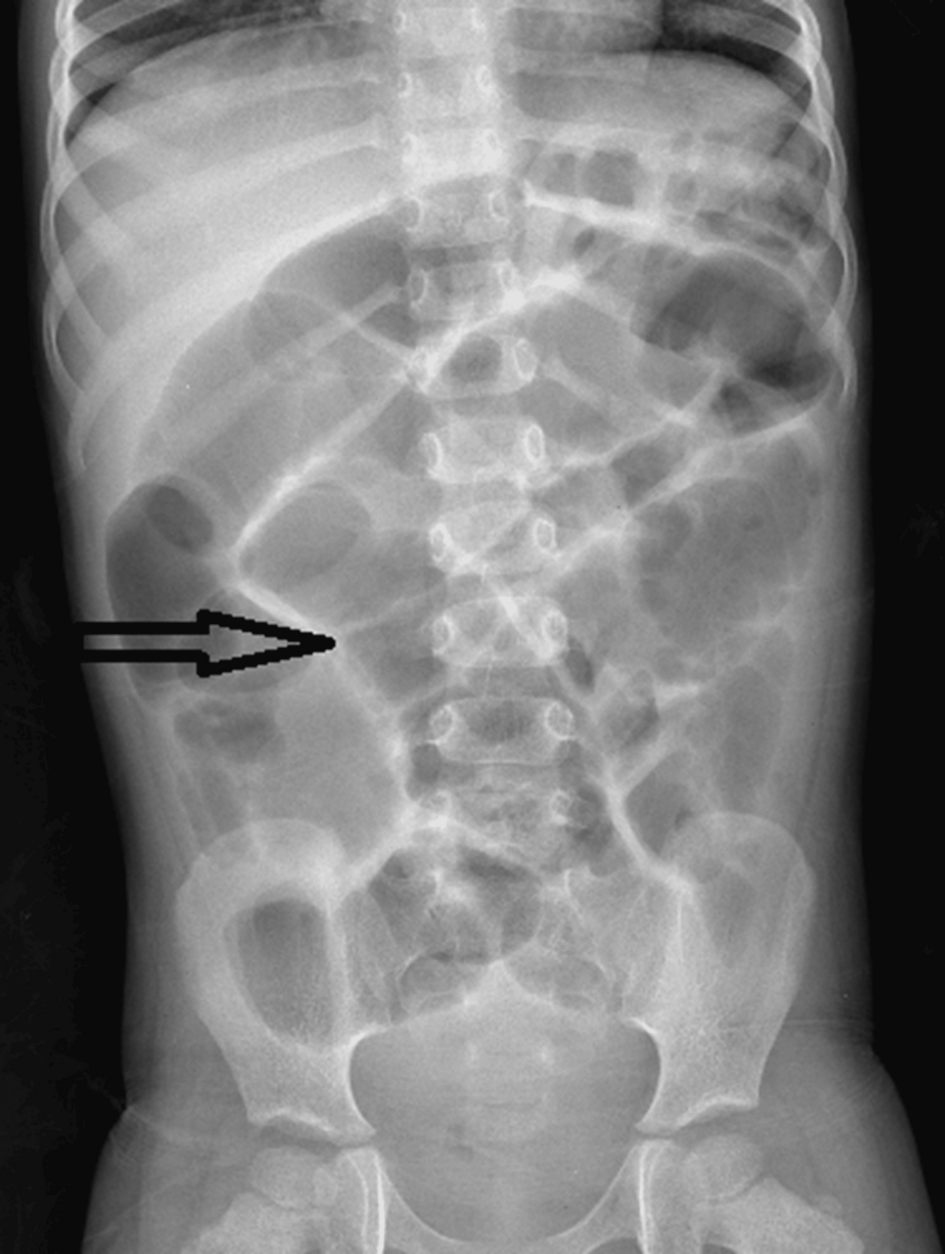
X-ray of the abdomen and pelvis showing dilated small bowel loops (arrowhead). Arrowhead showing dilated small bowel loops.
